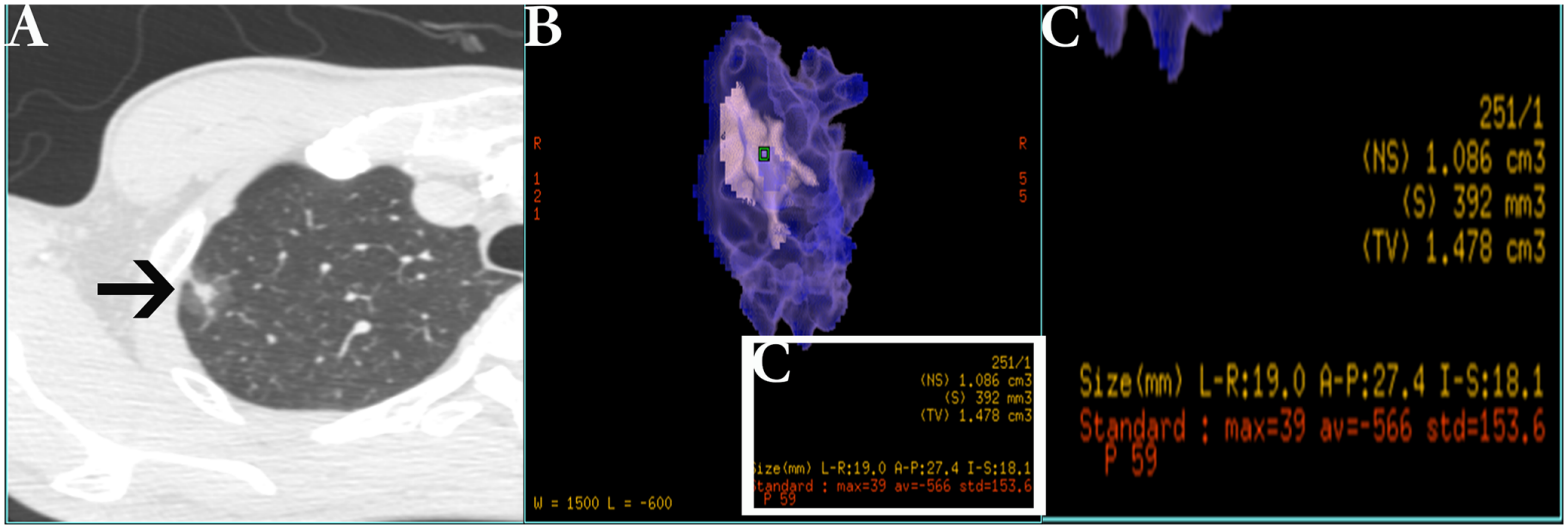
Example of nodule three-dimensional processed and measured on thin-section helical computed tomography (CT) images. (A) Typical ground-glass opacity (GGO, black arrow) nodule on high-resolution CT. (B) The software automatically processed and measured the nodule that be placed a marker (green square) on it. (C) The magnified measurement list of relative image parameters from picture B including diameter, total volume (TV), the maximum CT value (MAX), average CT value (AVG) and standard deviation of CT value (STD).
